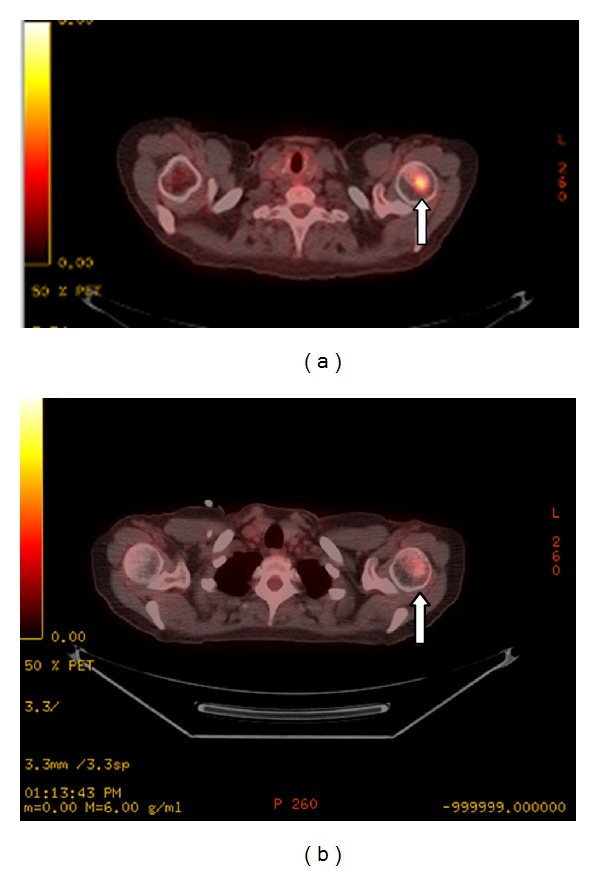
Axial PET-CT fusion image demonstrates an FDG-avid lesion in the left humeral head, consistent with a metastasis, labeled with an arrow (a). Postchemotherapy PET-CT axial image obtained just a few months later demonstrates complete metabolic response to the left humeral head lesion, labelled with an arrow (b).
